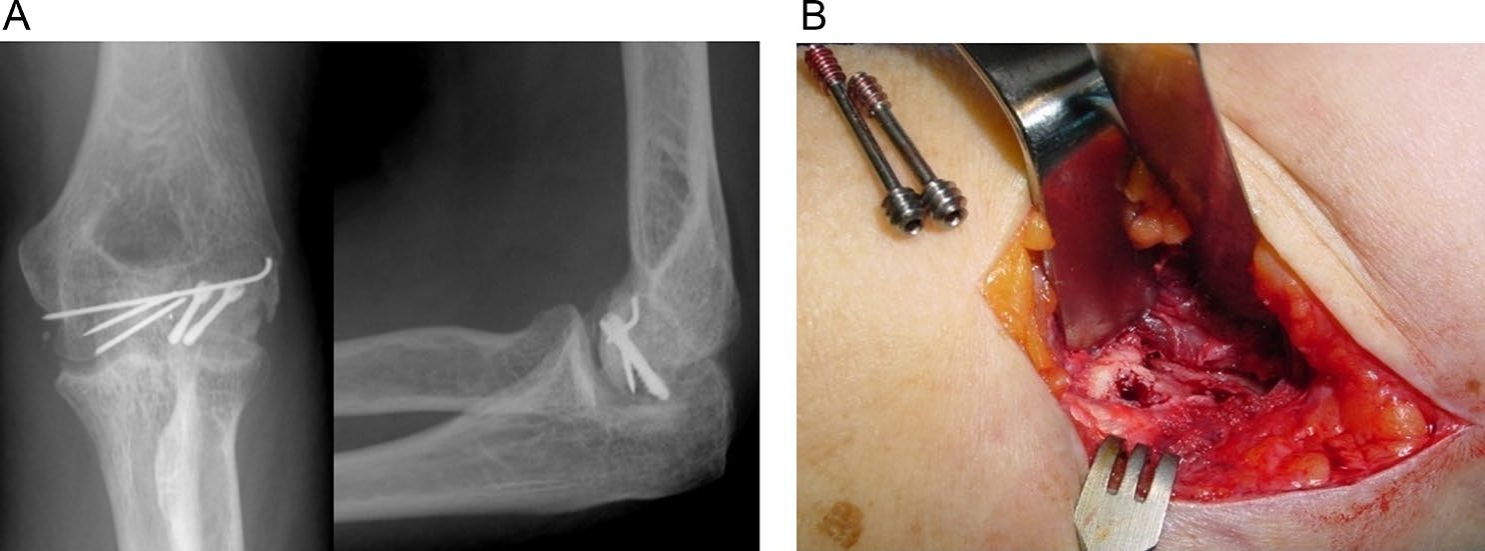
(A) x-ray showing partial necrosis of capitellum at 3months postoperatively. (B) hardware removal and arthrolysis at 1 year postoperatively
